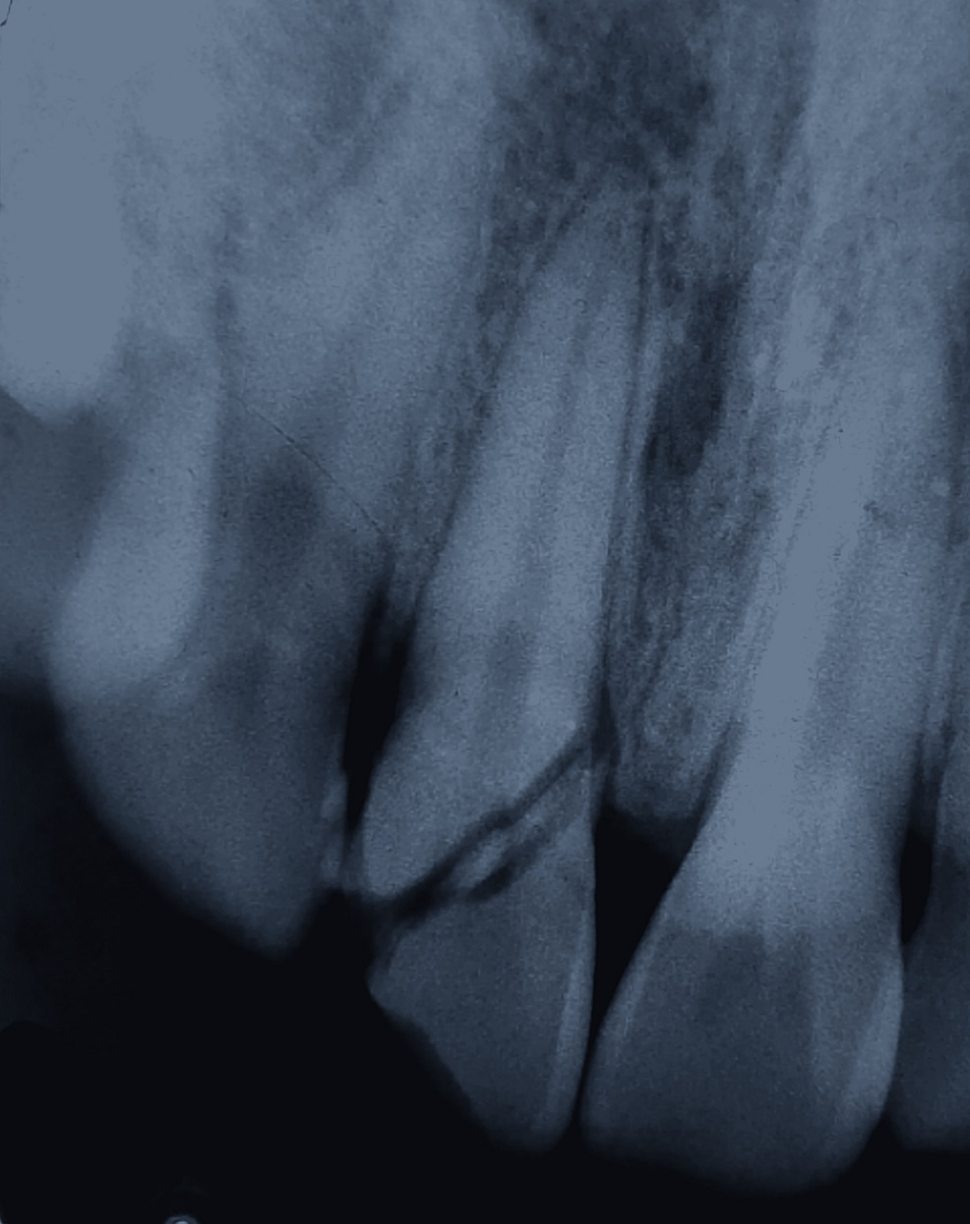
Intraoral periapical radiograph with respect to 11
